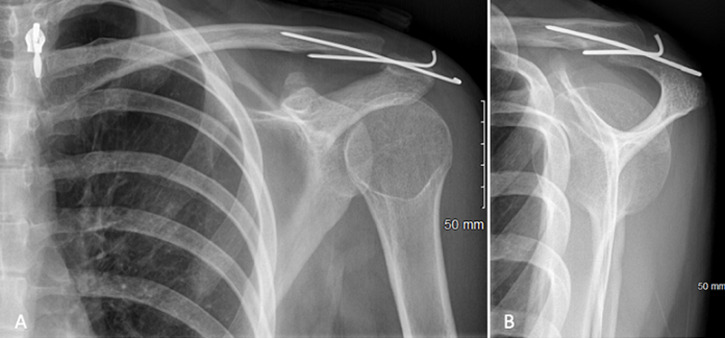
A) post-operative X-rays on day 1, AP; B) and lateral view
